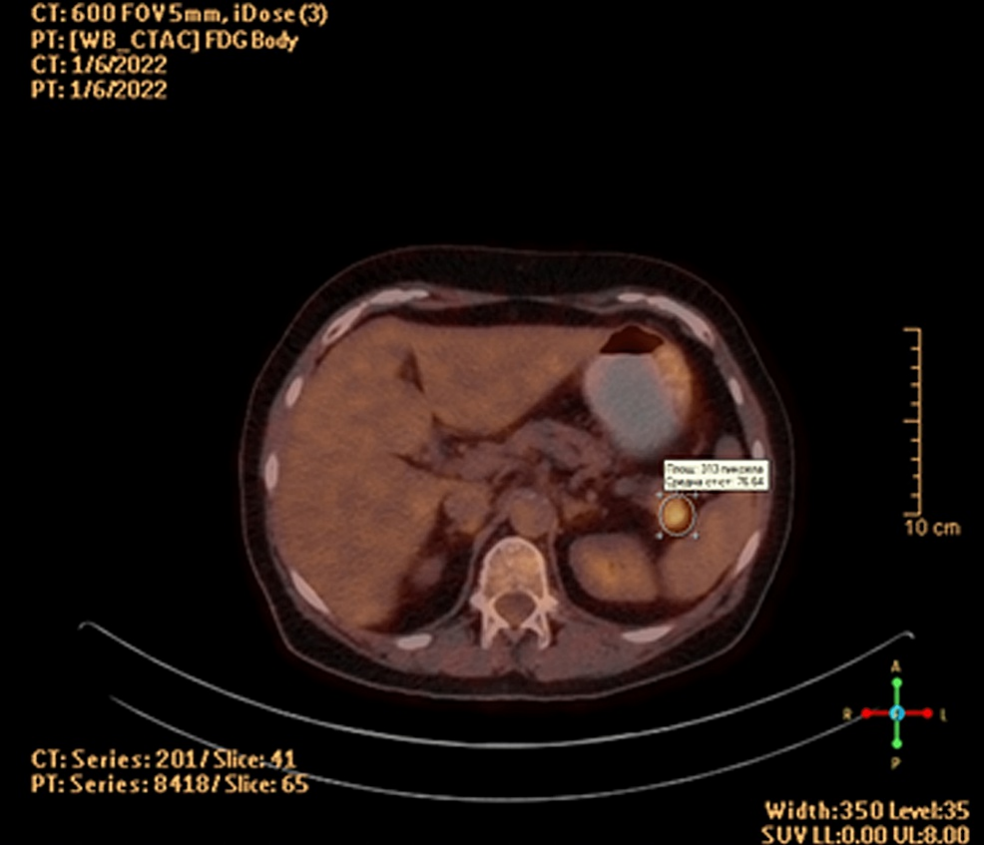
PET/CT of the patient PET/CT: positron emission tomography/computed tomography
